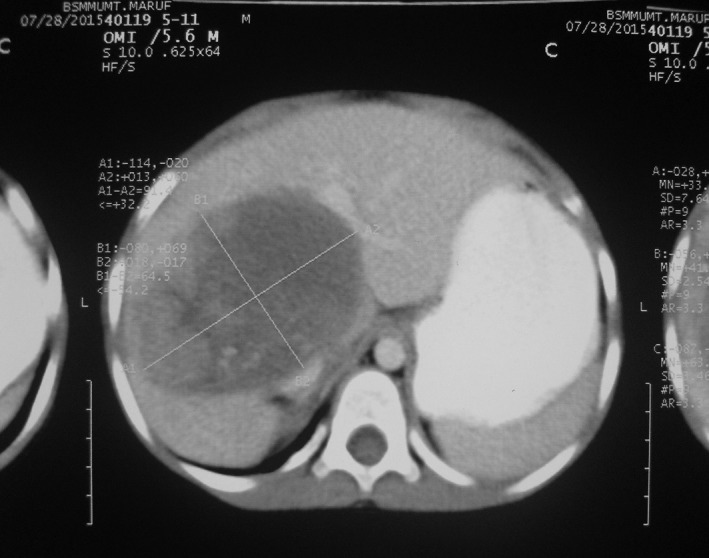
CT scan abdomen shows rounded hypodense area in right lobe of liver.
